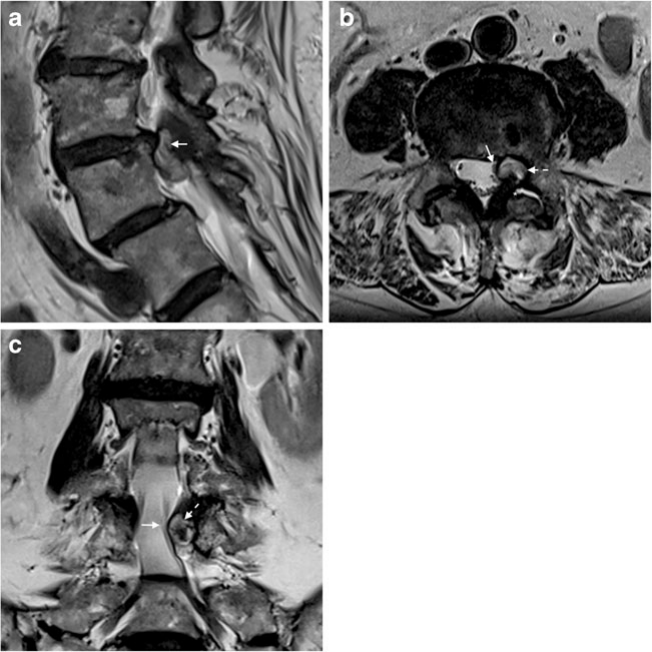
a-c. Lumbar facet synovial cyst in an 82-year-old woman presenting with subacute left lumbar radiculopathy and neurogenic claudication. Sagittal T2-weighted MRI (a) shows a slightly hyperintense cystic lesion posteriorly to the L3/L4 disc (arrow), as well as grade 1 degenerative spondylolisthesis at L4/L5. The axial view (b) clearly demonstrates the extradural location of the lesion (dashed arrow) arising from the left L3/L4 degenerated facet joint, which presents synovial effusion (asterisk). Note in both axial and coronal (c) views the displacement of the thecal sac and the left L4 nerve root (arrows) toward the right, due to compression by the cyst (dashed arrows). The partial T2-hypointensity, more evident in image c, might correspond to high-protein content or previous internal bleeding
